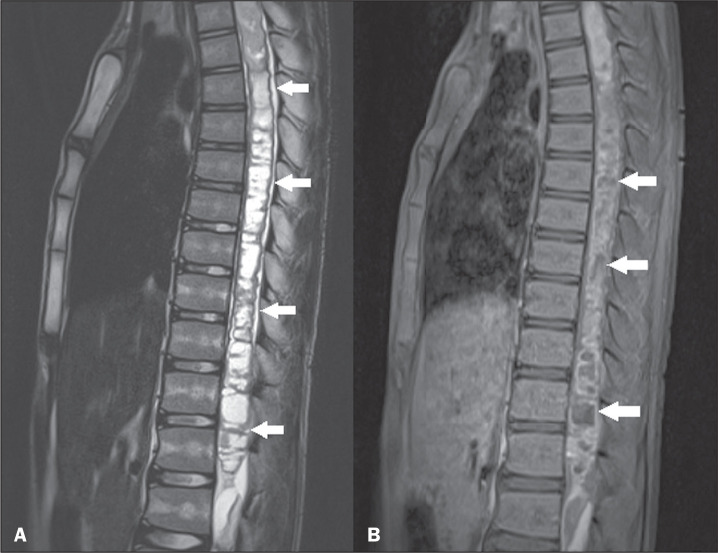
Ganglioglioma. Sagittal MRI scans. A: T2-weighted sequence showing a
